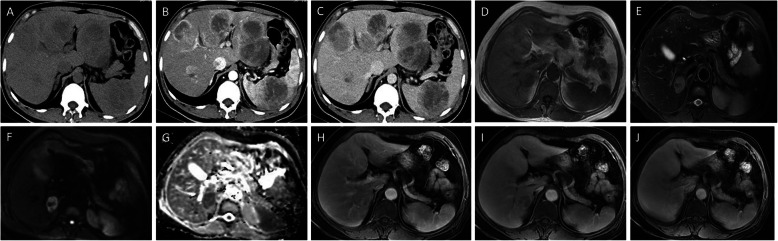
Typical cases of MHP. A 31-year-old male patient. The unenhanced CT image shows multiple hypodense hepatic lesions and spleen metastasis with ill-defined margins (a), heterogeneous marginal enhancement on the arterial phase (b), and progressive centripetal enhancement without full filling on the portal venous phase (c). A 55-year-old female patient with PHA. The MR images show hypointense lesions at the right hepatic lobe on T1WI (d), hyperintensity on T2WI (e), hyperintensity on DWI (F), hypointensity on the ADC map (g), slightly marginal enhancement on the arterial phase (H), patchy filling enhancement on the portal venous phase (i) and progressive, gradual filling enhancement on the delayed phase (j)
